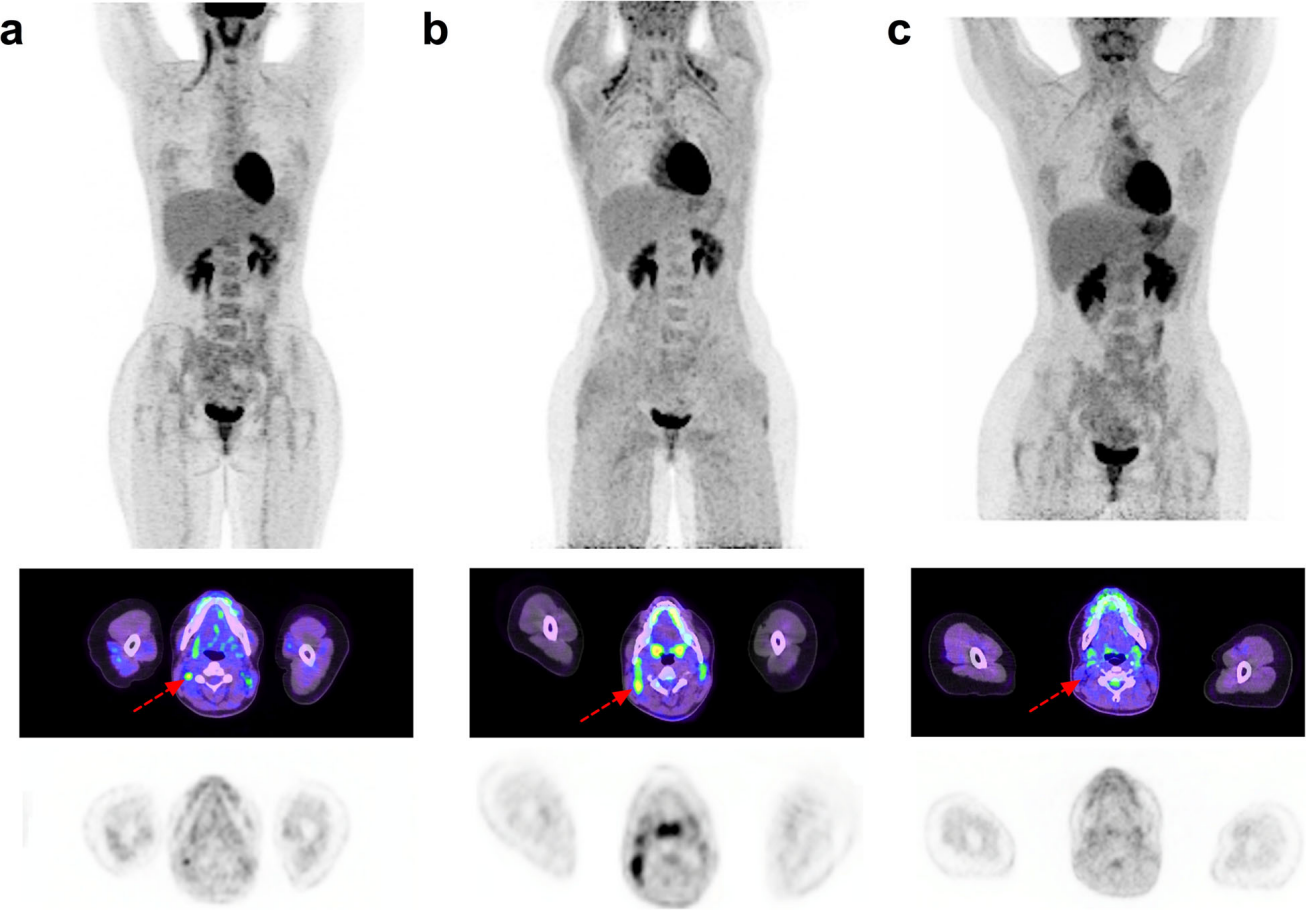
31-year-old female with Hodgkin Lymphoma. From top to bottom, Maximum intensity projection (MIP) PET, fused axial PET/CT and stand-alone PET images: a June 2018: Baseline PET showing stage 2 disease in the right neck. b January 2020: End-of-treatment PET considered non-diagnostic due to BAT activation in sites involved on baseline scan. c March 2020: follow-up PET examination. The patient received 40 mg of propranolol 1H prior to FDG injection. BAT activation is no longer visible and the patient is considered in complete metabolic response (Deauville score 2) with mediastinal uptake interpreted as thymic hyperplasia
